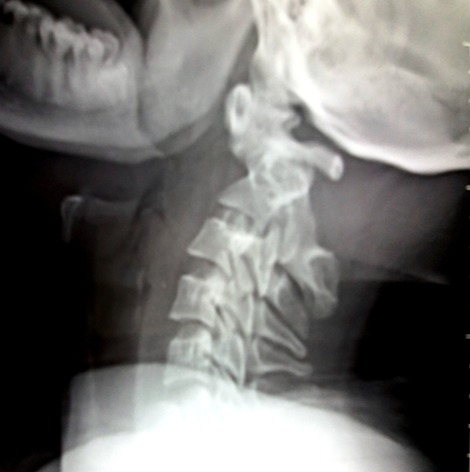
Cervical spine X-ray showing C1-C2 dislocation with posterior displacement of the odontoid process
